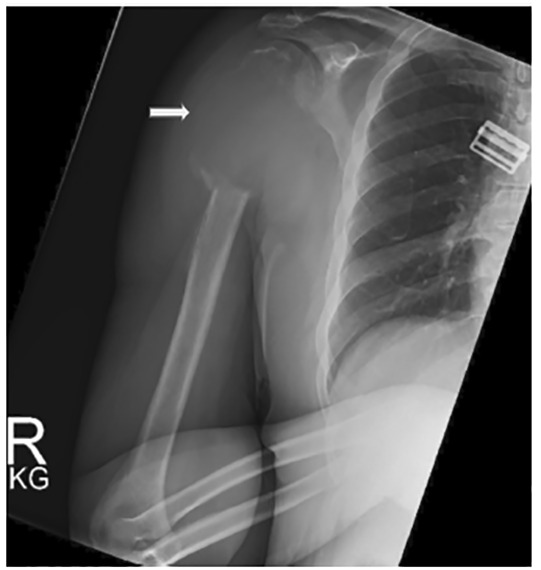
Right humerus radiograph demonstrates an area of presumed soft-tissue mass causing bony destruction of the right proximal humerus (arrow) three months later.
